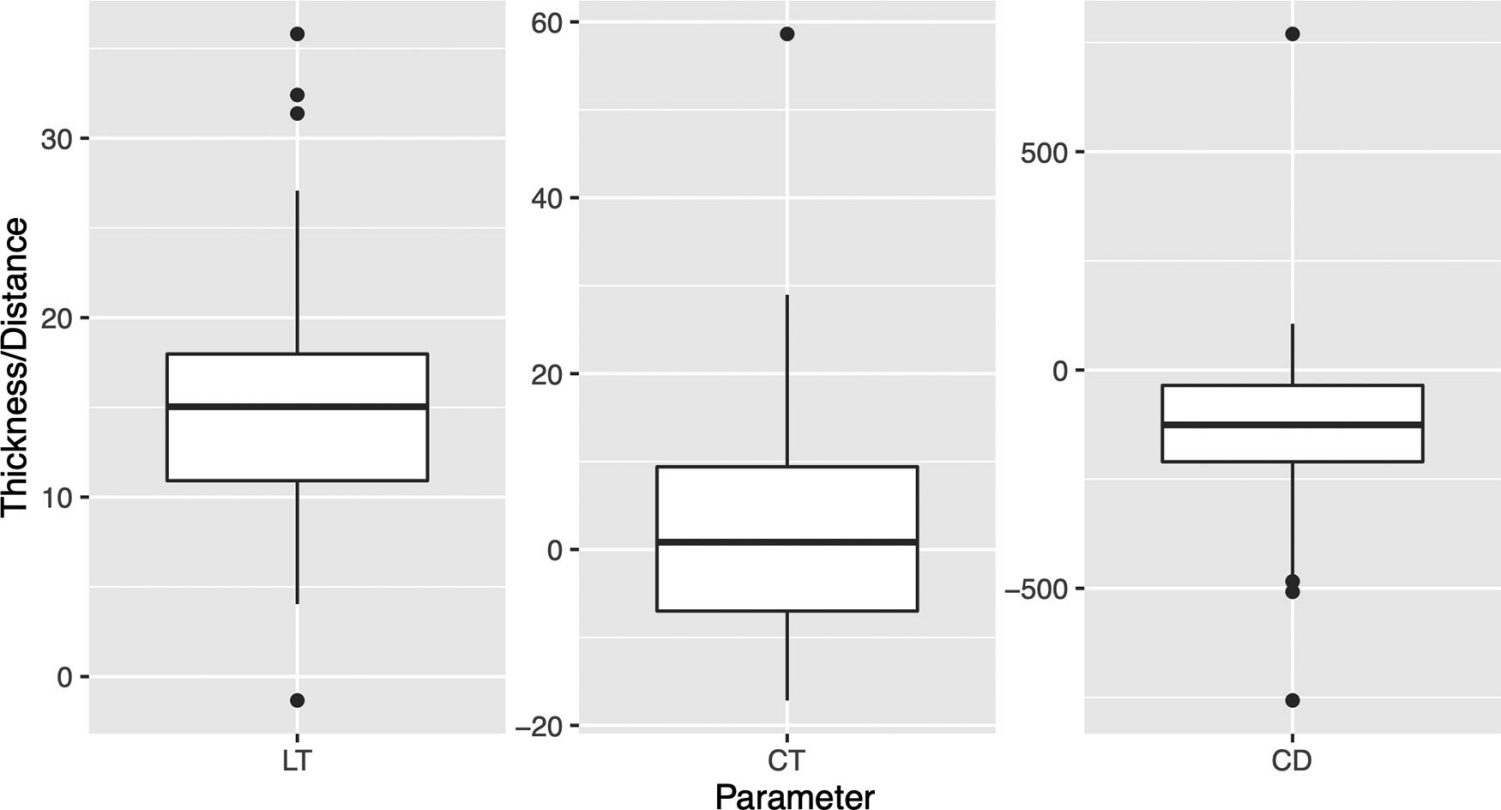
Deviation from calculated LT, CT, and CD.
